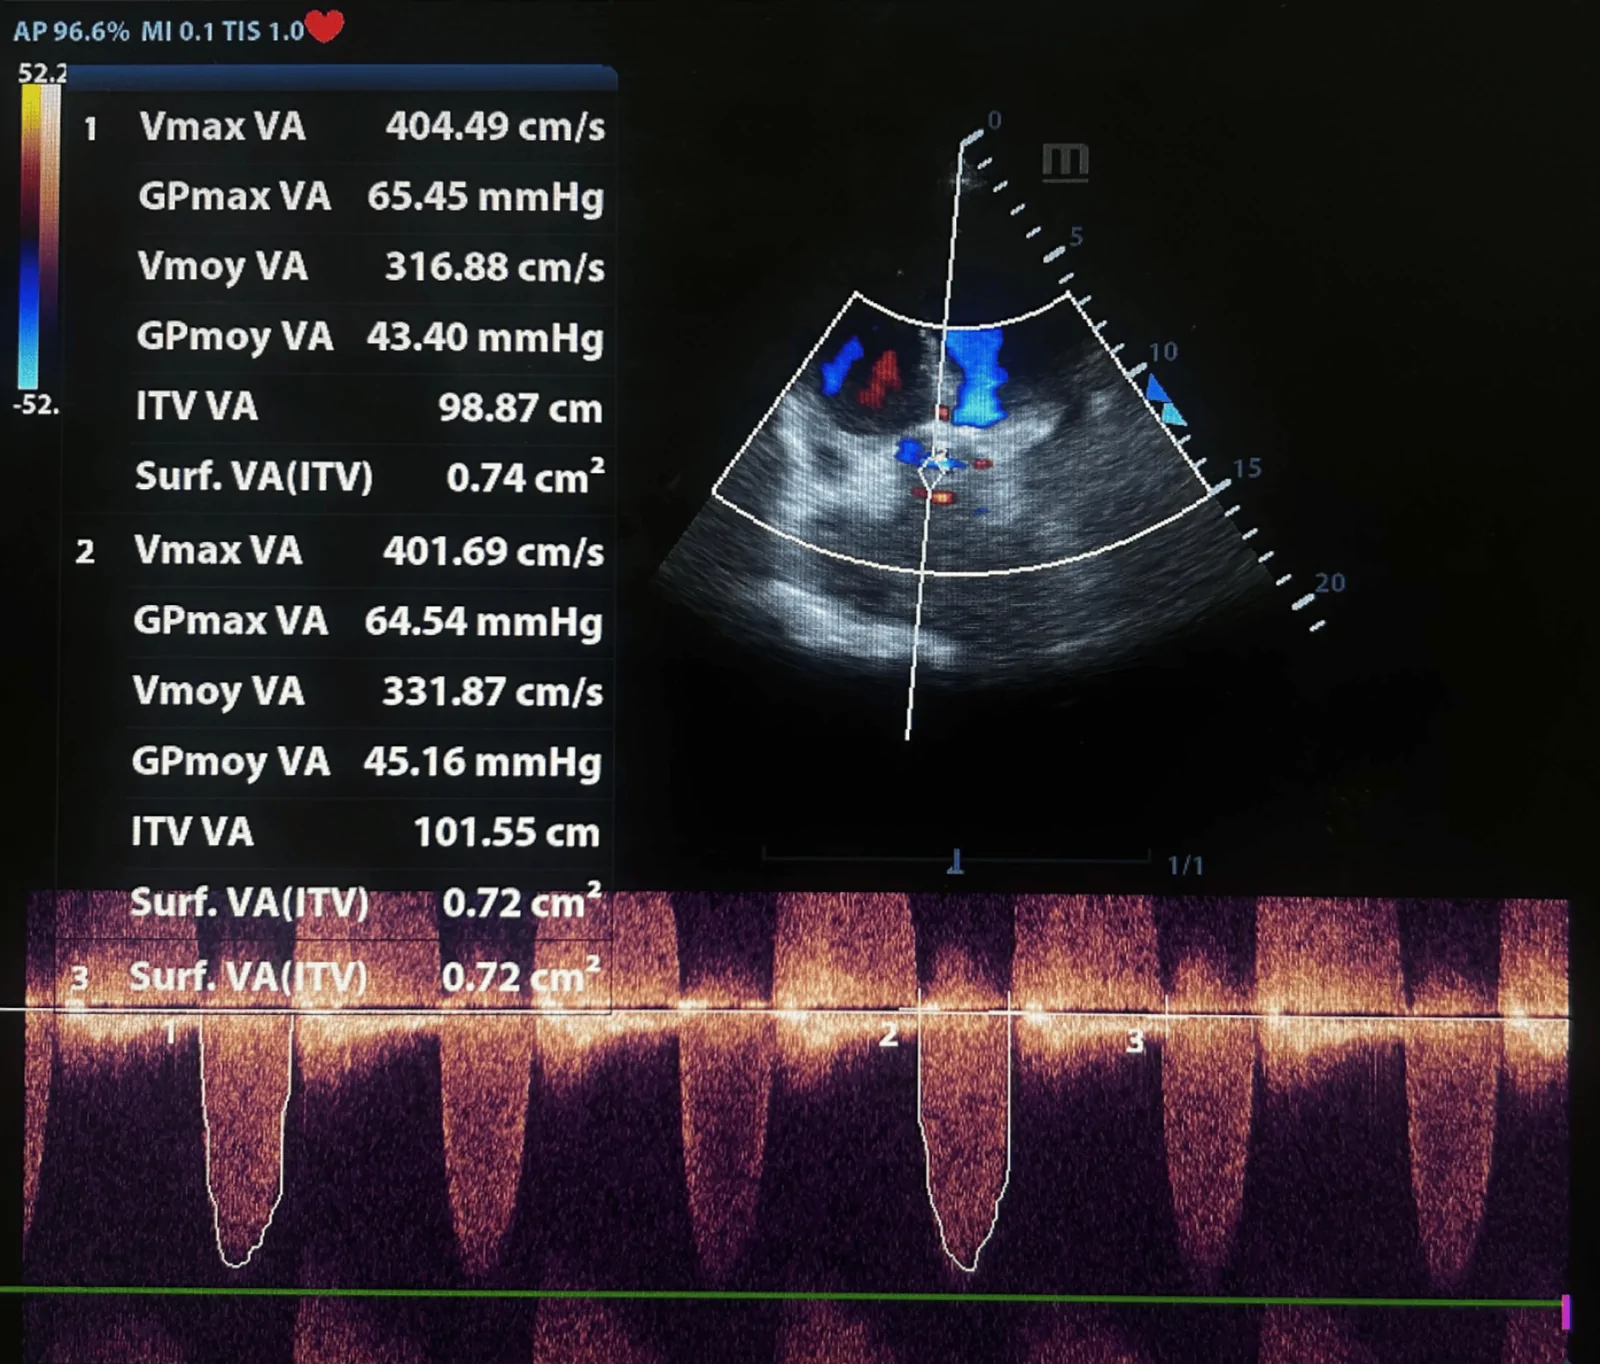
Echocardiographic assessment of aortic stenosis in Case 1. Continuous-wave Doppler echocardiography demonstrating severe aortic stenosis. Repeated measurements showed peak aortic jet velocities of 4.02-4.04 m/s, mean transvalvular gradients of 43.40-45.16 mmHg, and calculated aortic valve areas of 0.72-0.74 cm².
